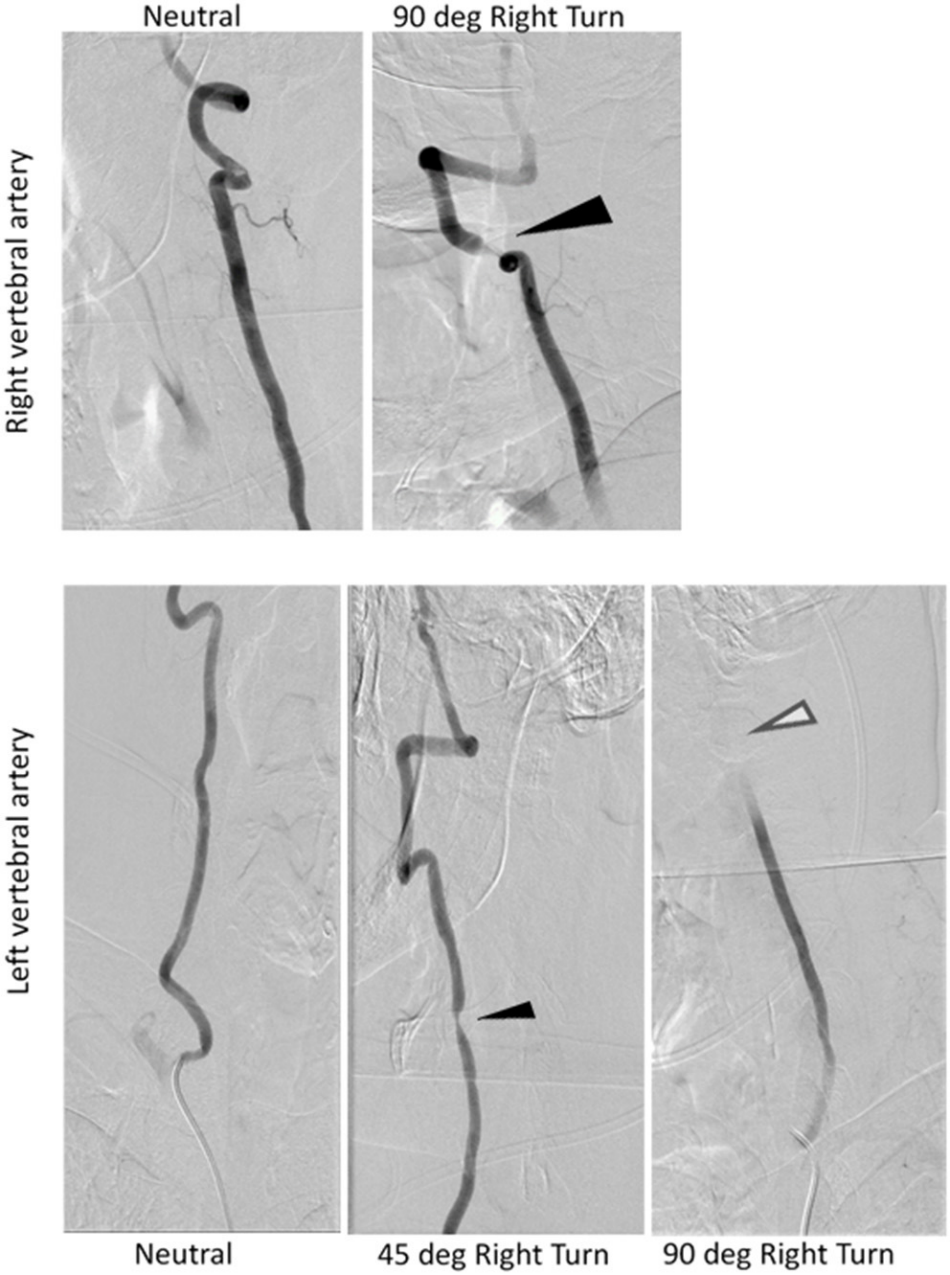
Radiograph evidence confirming severe stenosis of right and left vertebral arteries during rightward head rotation. Dark arrows reflect partial stenosis, white arrow reflects absent perfusion from complete stenosis.
